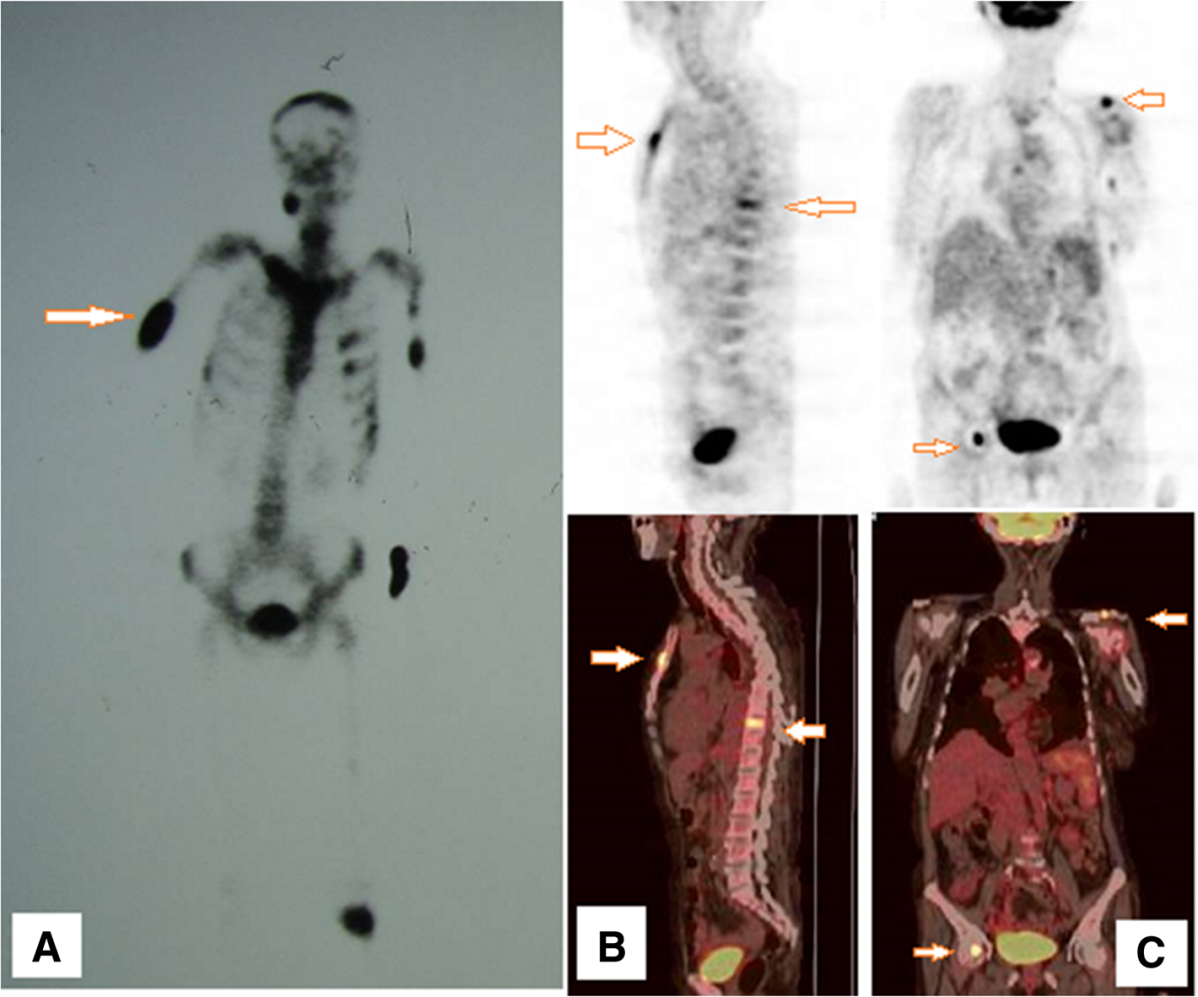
Emission computed tomography (CT) showing increased radioactive concentration in the brustwirbels, sternum, ilium, and left clavicle (A). Positron emission tomography/CT showed generalized lymphadenopathy, increased whole-body bone metabolic activity, and generalized skeletal destruction. The ^18^ F-FDG standard uptake value was increased in the whole skeleton and was 5.1 in the sternum (B), and 6.1 in the femur and clavicle (C).
